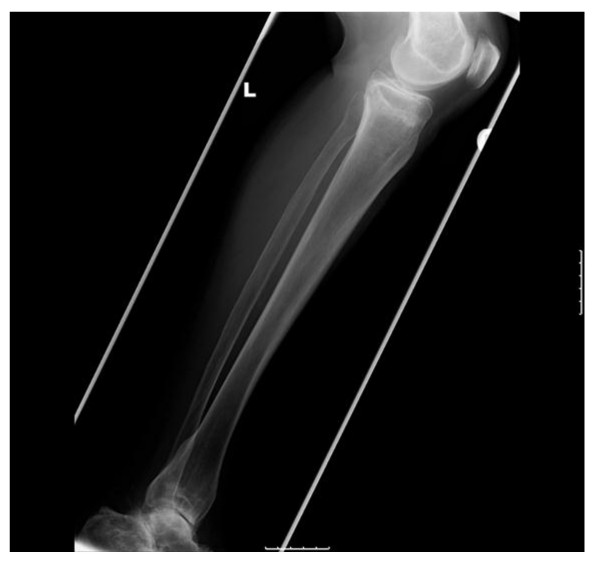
Lateral radiograph of the left tibia six months after diagnosis with resolution of symptoms and healed fractures.
